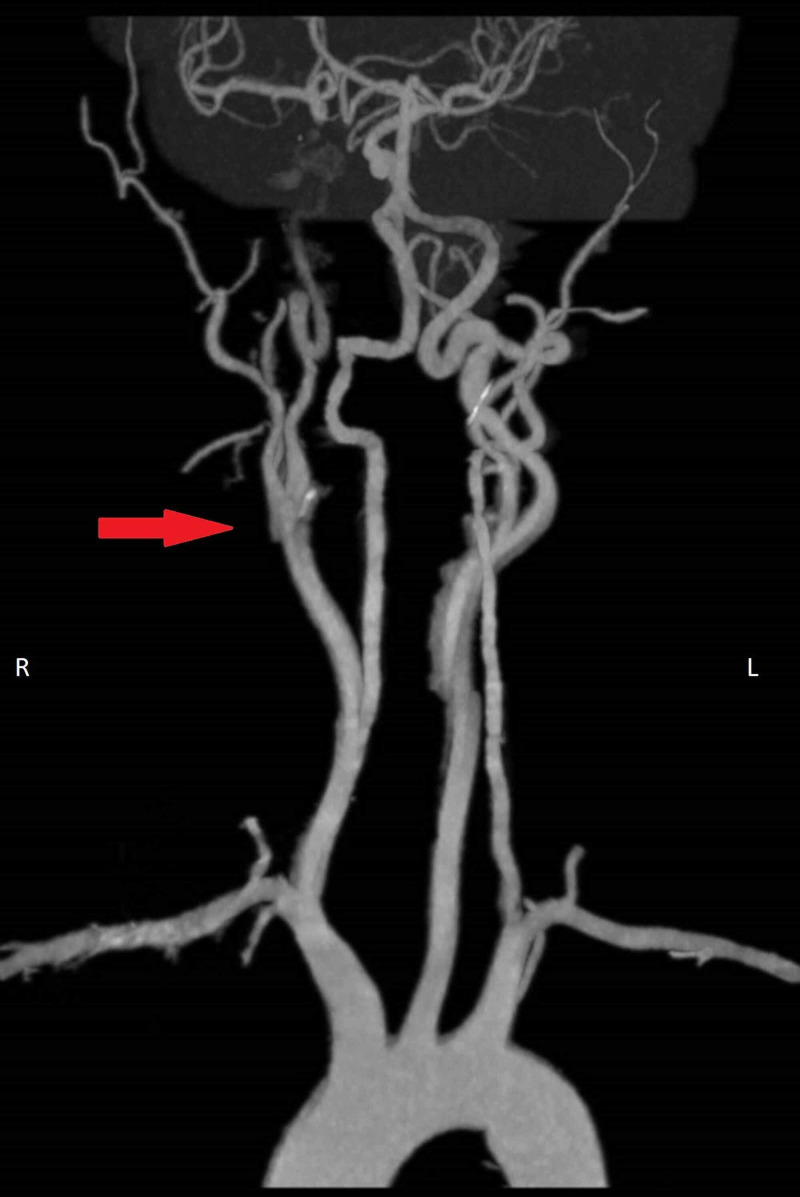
CT angiogram demonstrating severe attenuation of right internal carotid artery (ICA) just beyond take-off and poor opacification up to cavernous segment. Motion artefacts degrade quality of picture.
